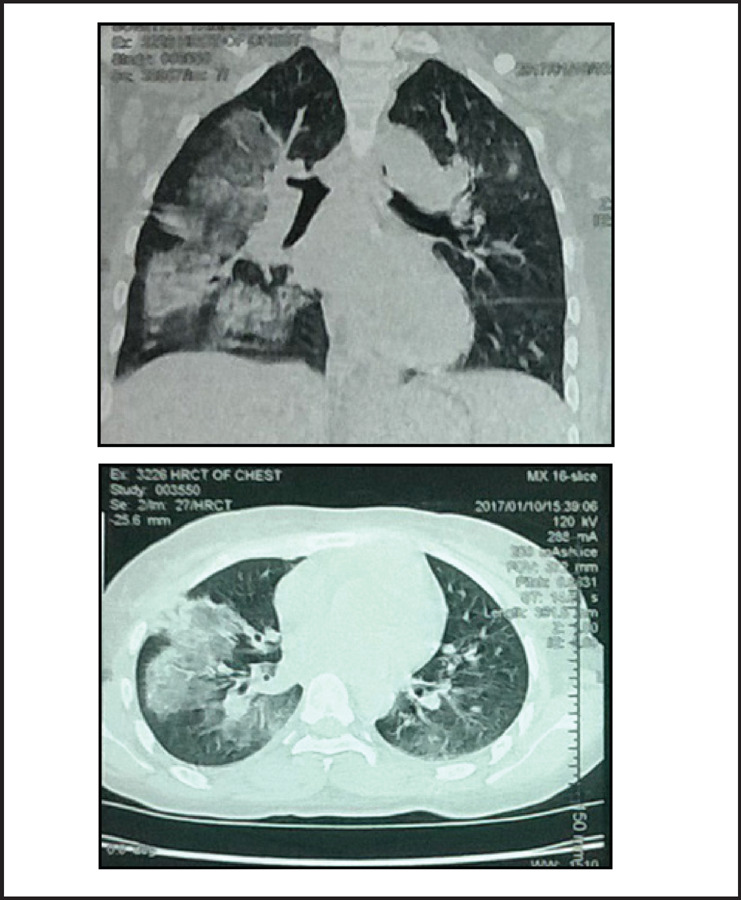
HRCT chest showing bilateral areas of consolidation and ground glass opacities, more marked on the right side.
